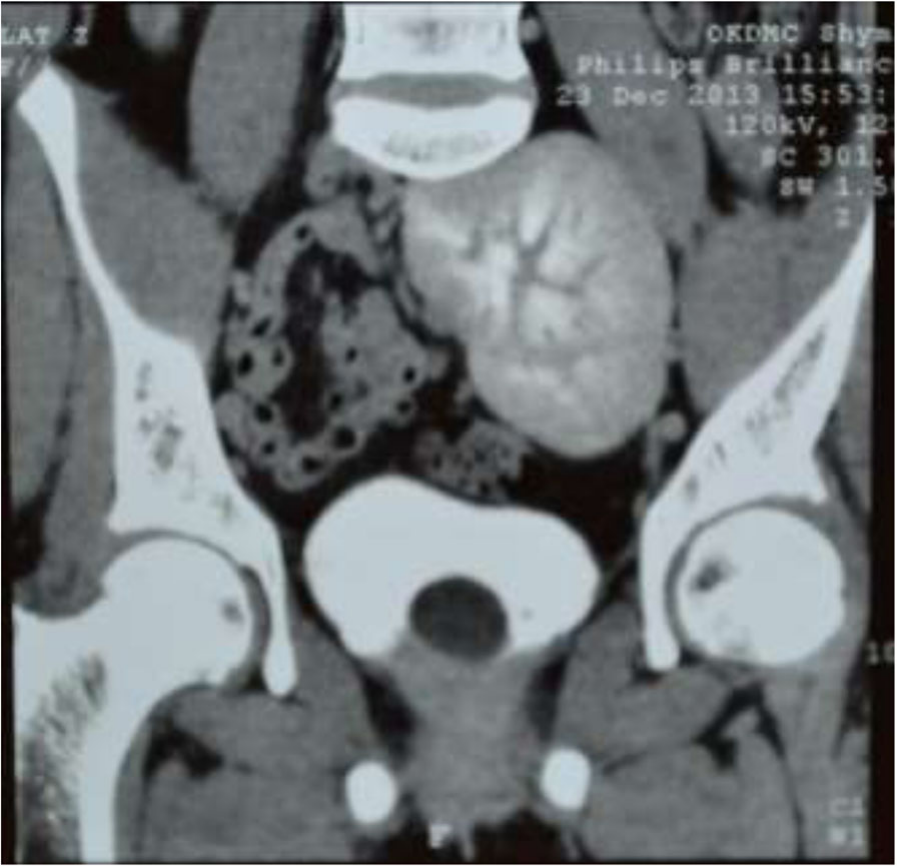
A contrast media computed tomography scan shows agenesis of the right kidney. Pelvic ectopy of the left kidney, 51.8 × 62.8 in size; on the right side of the urinary bladder there is a cystic mass 24 × 17 mm in size (papilloma, ureterocele, mixed tumor). Agenesis of uterus and vagina (Mayer-Rokitansky-Küster-Hauser syndrome) are shown
